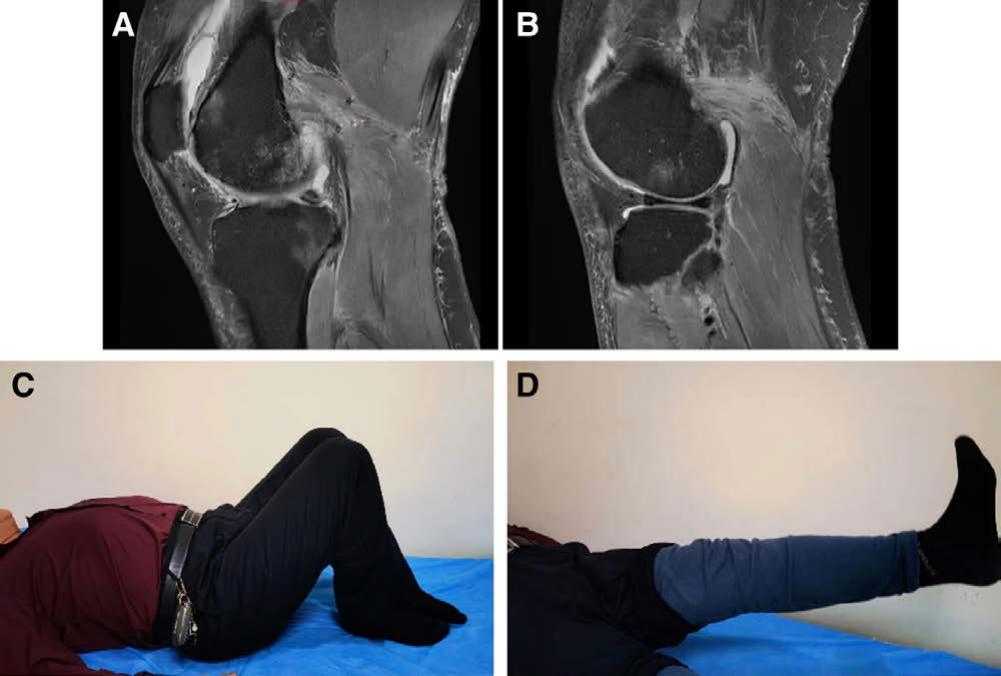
Follow-up examination 6 months after the first-stage surgery: (A) sagittal proton density magnetic resonance image of the medial head of gastrocnemius muscle (arrow), (B) sagittal proton density magnetic resonance image of the lateral head of gastrocnemius muscle (arrow), (C and D) the patient’s left knee hyperextension was significantly improved.
